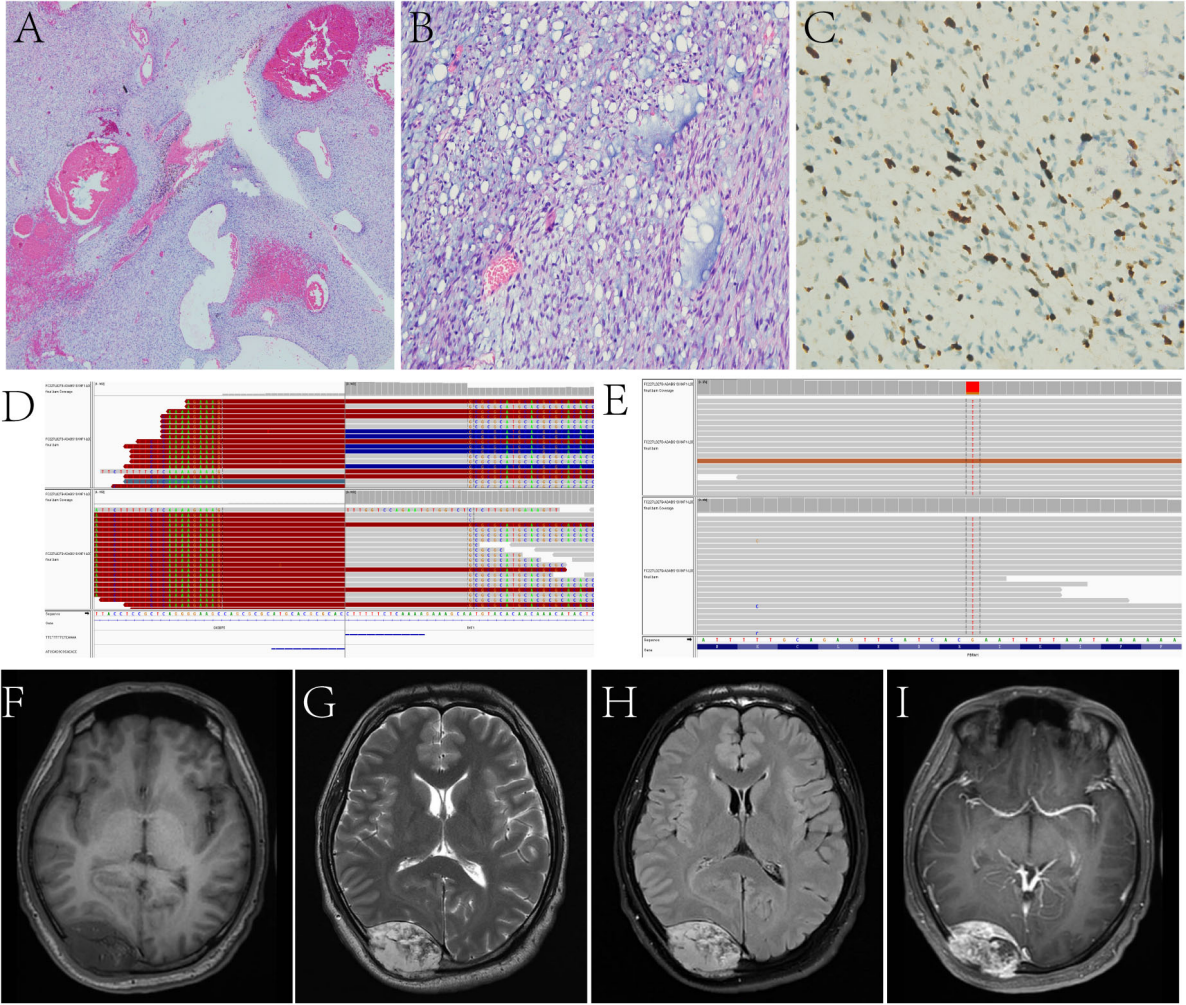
In Case 10, the tumor is arranged in bundles, the myxoid stroma is seen between the tumor cells, and dilated blood vessels are seen inside the tumor (A, B). Ki-67 LI is about 10% (C). Target NGS sequencing finds the SH3BP5::RAF1 gene fusion (D) and the mutation of PBRM1 (E). A fusiform solid lesion is sited in the right occipital region and can be seen in the extradural area and invades the skull, T1WI (F) shows hypointensity, T2WI (G) and T2 FLAIR (H) shows hyperintensity, and contrast-enhanced T1WI (I) shows inhomogeneous enhancement.
